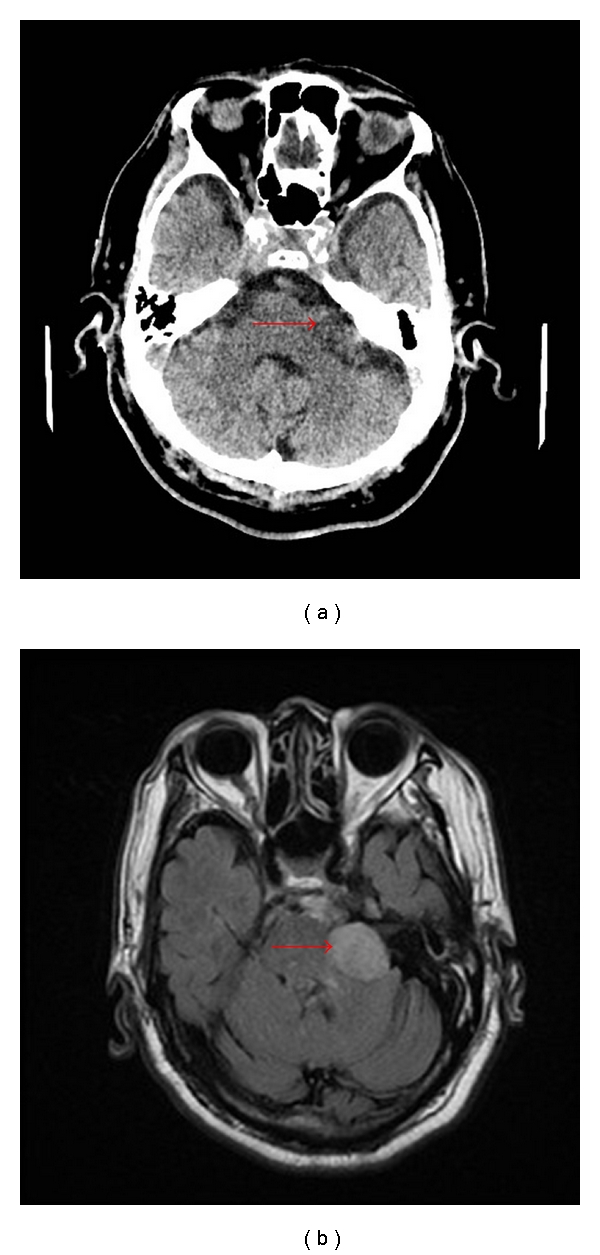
(a) Non-contrast head CT showing a heterogeneous lobulated mass within the left cerebellopontine angle (arrow). (b) Followup brain MRI in same patient 3 days later.
